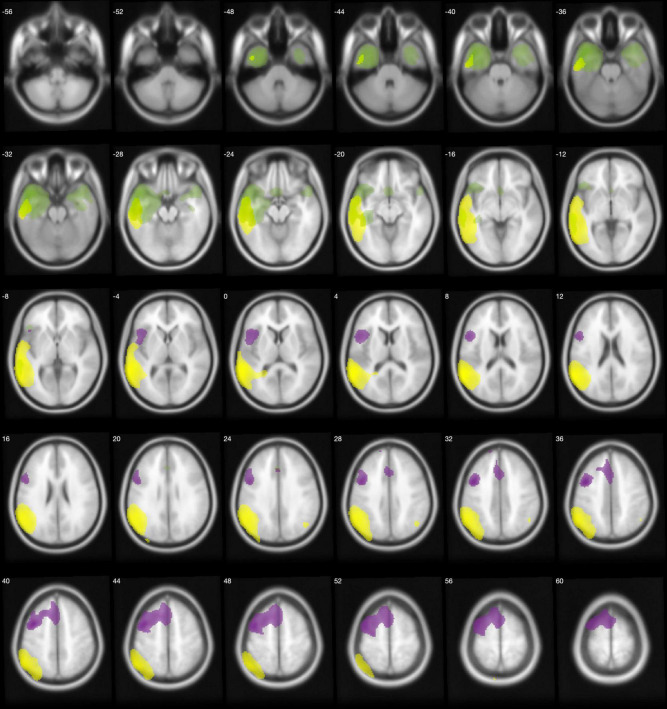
Regions with lower metabolism in the PPA variants displayed on an MRI template. nfPPA (violet), svPPA (green), and lvPPA (yellow) were compared with healthy controls using a 2-sample *t*-test with a family-wise error corrected *p* < 0.05. Images are shown using neurological orientation.
